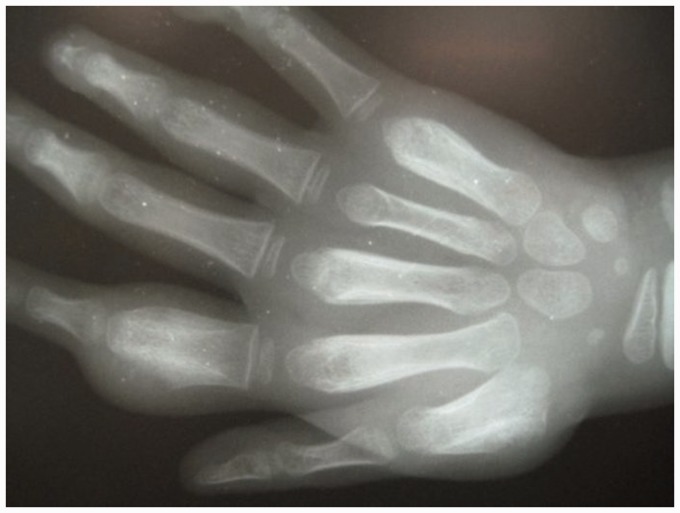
Secondary yaws: radiographic evidence of osteoperiostitis. Copyright Oriol Mitjà.
